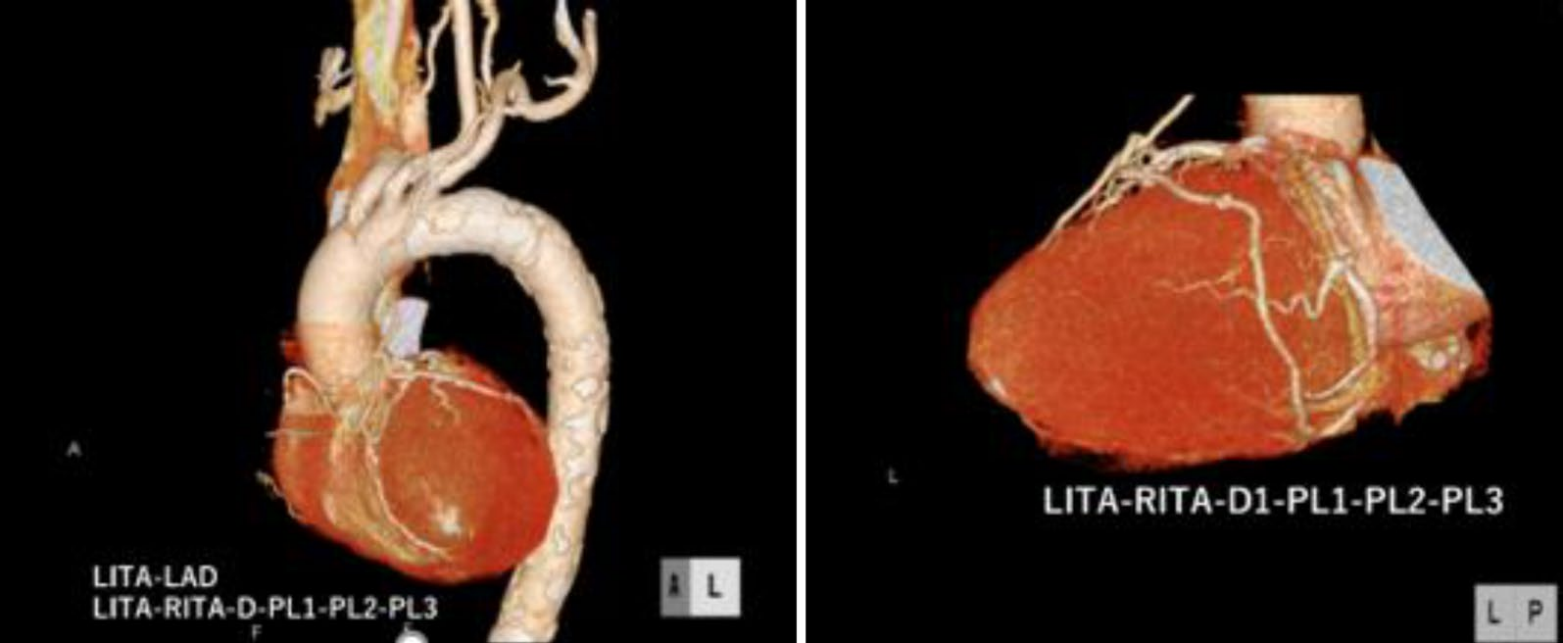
Postoperative three-dimensional computed tomography (3D-CT) in Case 2. All grafts are patent
